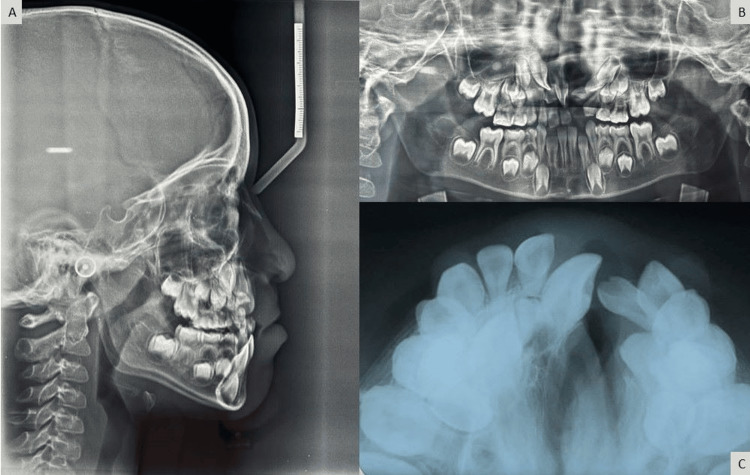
Post-expansion radiographs: (A) lateral cephalogram, (B) orthopantomogram (OPG), and (C) maxillary occlusal
